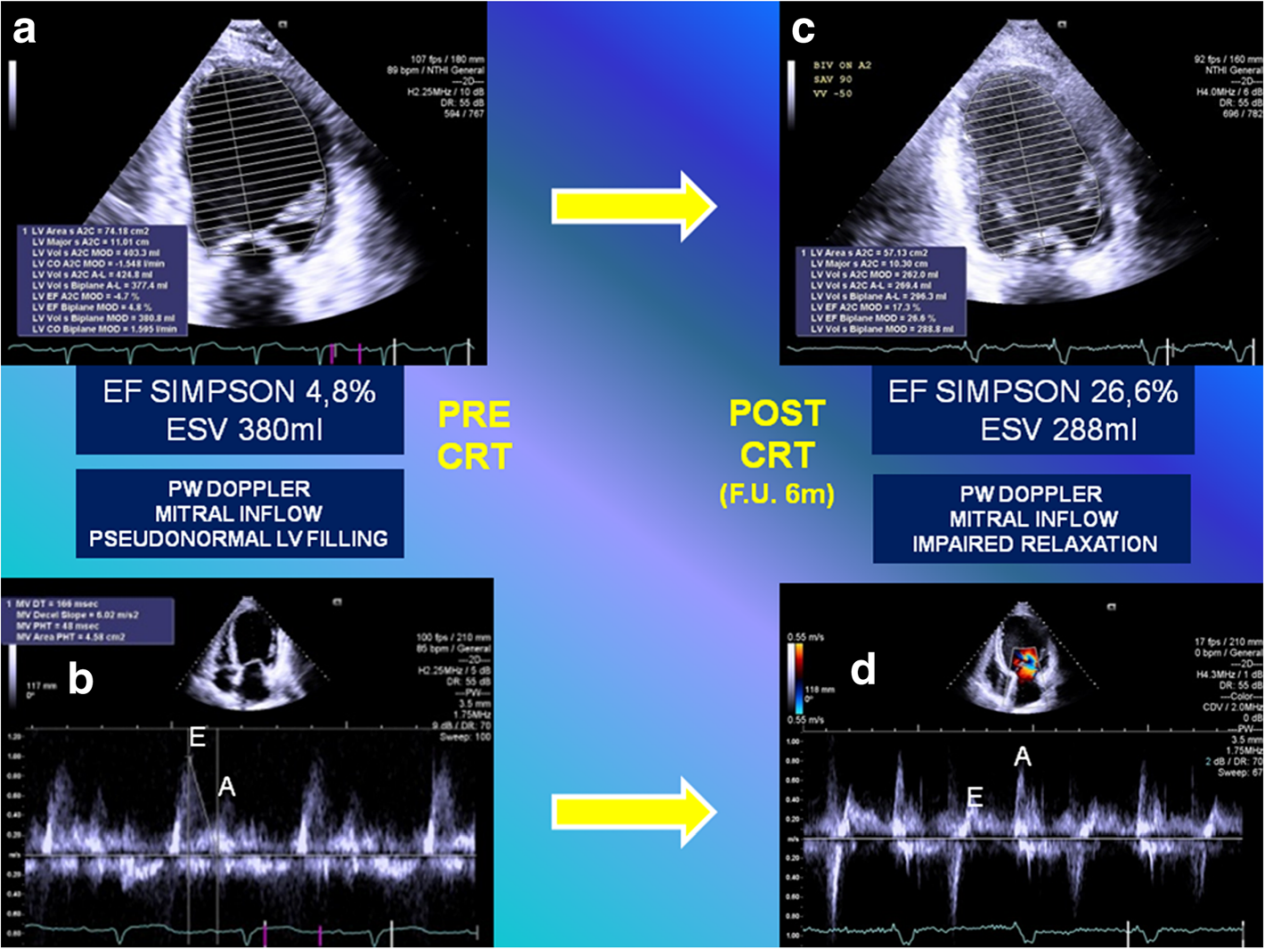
Left side*: a Dilated left ventricle with an end-systolic volume of 380 ml, and an ejection fraction of 4.8% as was measured by the modified Simpson’s method pre-implant. b Pulsed wave Doppler mitral inflow pattern showing advanced diastolic dysfunction (deceleration time (*DT*) of early filling velocity, 165 ms). *Right side*: c Dilated left ventricle with reduced end-systolic volume of 288 ml and improved ejection fraction of 26.6% as was measured by the modified Simpson’s method post-implant at 6-month follow-up. d Pulsed wave Doppler mitral inflow pattern showing impaired relaxation. *CRT* cardiac resynchronization therapy, *EF* ejection fraction, *ESV* end-systolic volume, *F.U.* follow-up, *LV* left ventricle, *PW* pulsed wave
